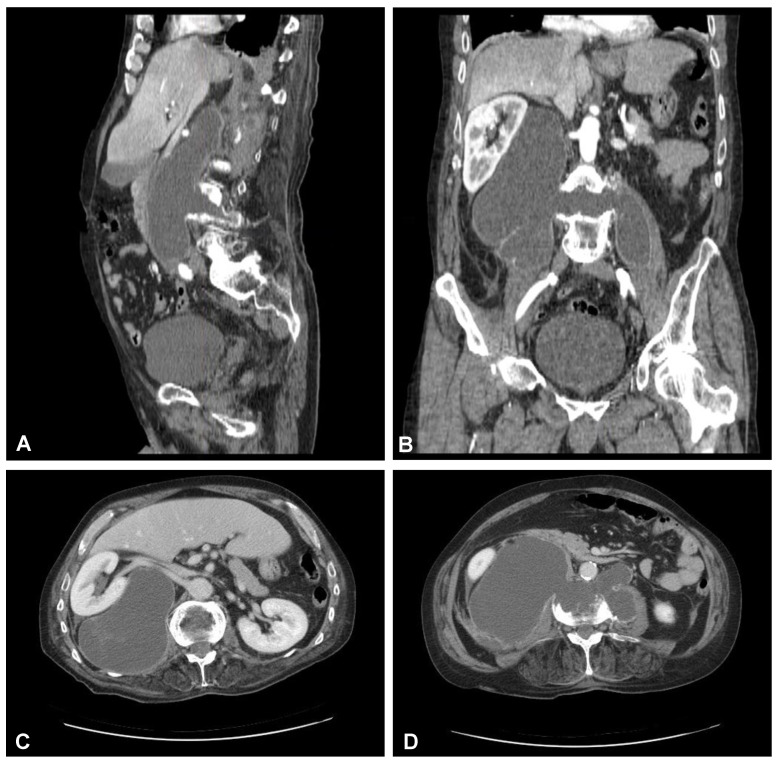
Preoperative sagittal (A), coronal (B), and axial (C,D) CT scans (contrast enhanced) of the lumbar spine demonstrating hypodense area with enhancing wall at the L3/L4 intervertebral disc space communicating with both psoas major muscles forming massive well-defined fluid-like collection in the right retroperitoneum.
